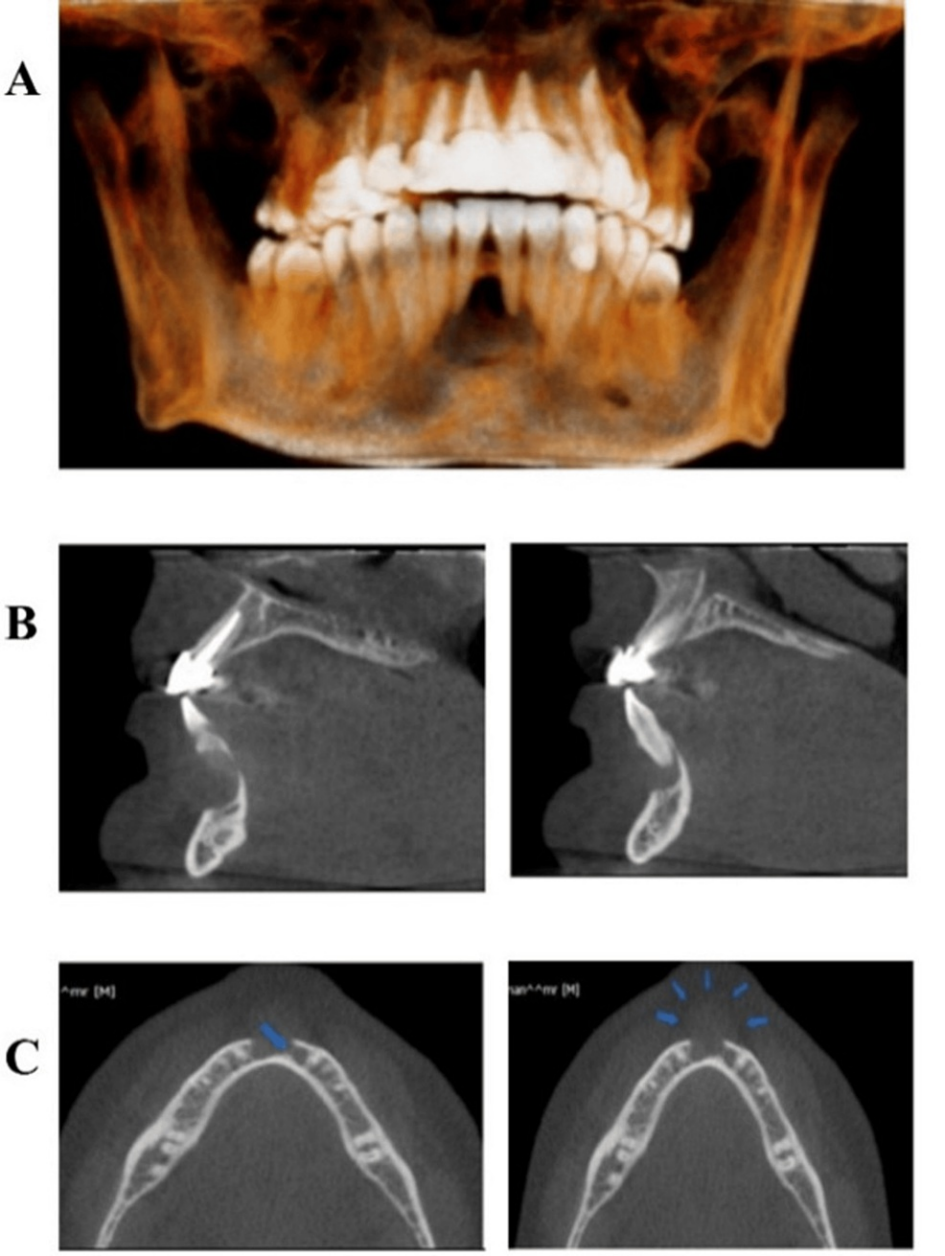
(A) Cone-beam computed tomography (CBCT) (Reconstructed). (B) Lateral view. (C) Axial view The imaging procedures detected the presence of a well-demarcated, non-corticated, pear-shaped radiolucent lesion in the anterior region of the mandible, extending from tooth #33 to tooth #43 (Figure 2A). The lesion caused the slight separation of roots of the lower central incisors, breached the buccal plate of bone, and thinned and eroded the lingual plate of bone (Figure 2B). There is evidence of faint septation within the lesion (Figure 2C). The soft tissue component of the lesion extended to the overlying labial soft tissues (Figure 2C). Based on the observed location and behavioral patterns, central giant cell granuloma is the most likely diagnosis. However, inflammatory cysts and keratocysts cannot be excluded.
